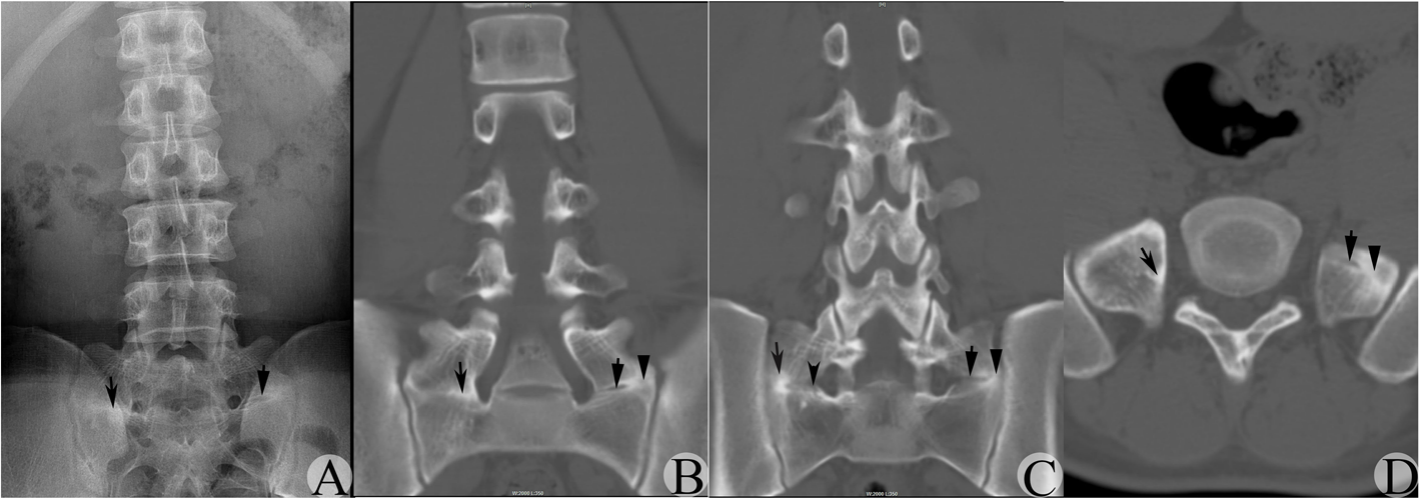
A suspected type IIb MA-LSTV judged by AP-LPR was verified to be type IIIb by CT-CRI. a AP-LPR image showing the suspected JLS existed bilaterally, and suspected JLS on the right side was narrower than that on the left side in the craniocaudal direction. Arrow indicates the suspected JLS on the left side; concave arrow indicates the suspected JLS on the right side. b CT-CRI showing the BUS was found in most CT-CRIs, while vague RSB was reserved at the medial region on the right side. Meanwhile, the intermittent JLS and BUS appeared simultaneously on the left side. Arrow, arrowhead and concave arrow indicates the JLS, BUS with RSB on the left side, and the BUS with RSB on the right side, respectively. c CT-CRI showing the detected JLS at the medial region in a few planes on the right side and the intermittent JLS and BUS appearing simultaneously at each plane on the left side. Arrow, arrowhead, concave arrow and concave arrowhead indicates the JLS and BUS on the left side, and the BUS and JLS on the right side, respectively. d Transverse CT images confirmed the JLS and BUS appeared in the same plane on the left side, and the BUS with RSB on the right side. Arrow, arrowhead and concave arrow indicates the JLS and BUS on the left side, and BUS with RSB on the right side, respectively
